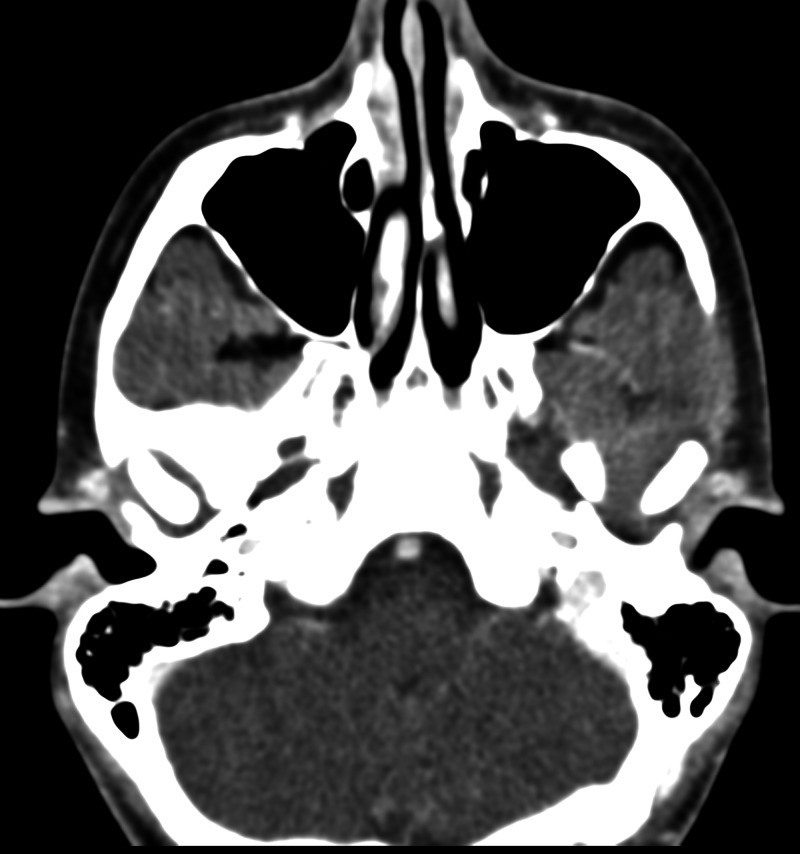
Axial non-contrast maxillofacial computed tomography (CT) (soft tissue window) demonstrates no soft tissue abnormality or mass
